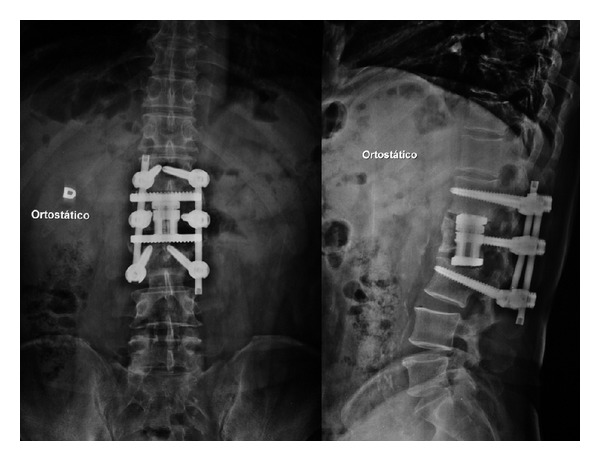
AP and lateral X-rays showing good coronal and sagittal alignments 12 months after surgery.
